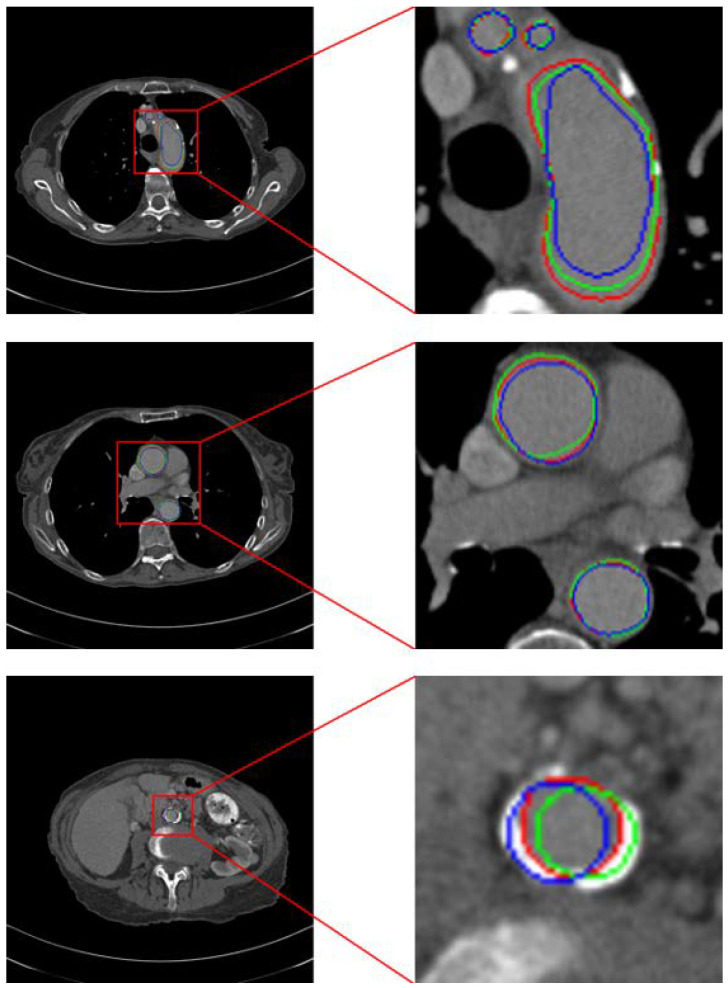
Location of aorta in CT images with similar serial numbers. The green circle is the location of the aorta in the previous CT image, the blue circle is its location in the next CT image, and the red circle indicates the location of the aorta in the central CT image.
